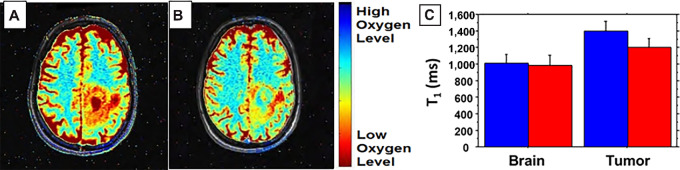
TOLD MRI of patient receiving 0.17 mL/kg DDFPe pretreatment (A) and posttreatment (B) shows reversal of tumor hypoxia. T1 of tumor (mean ± SD) was 1,454 ± 75 ms preadministration of DDFPe and decreased to 1,281 ± 77 ms after treatment. NMR relaxation measurements before and after infusion of DDFPe (C). NMR T1 measurements represent mean ± SD for the 4 patients encompassing tumor or contralateral normal brain imaged at 1.5 T. Baseline measurements (blue) were performed before administration of DDFPe, and postmeasurements (red) were made following intravenous administration of DDFPe, while patients breathed oxygen or carbogen following irradiation. Normal brain tissue showed no significant response (*P* = 0.70), while tumor showed significant change following administration of DDFPe and irradiation (*P* = 0.0059). Considering all measurements, tumor tissues had significantly longer T1 relaxation times than normal brain (*P* < 0.0001). At baseline brain T1 was significantly shorter than tumor (*P* < 0.0001) and this remained so after administering DDFPe (*P* = 0.0045).
